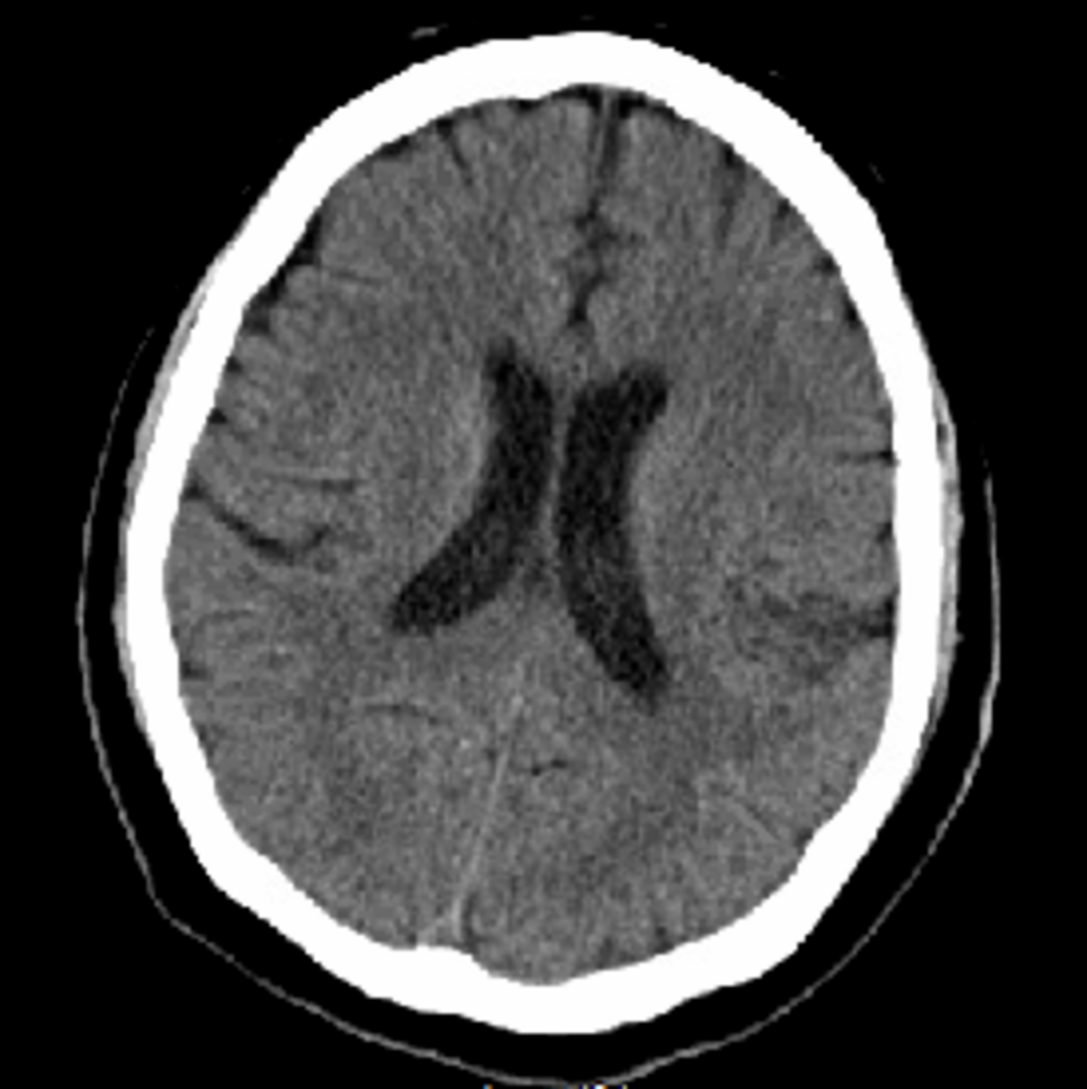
Computed tomography of the head without contrast did not reveal acute intracranial pathology.
